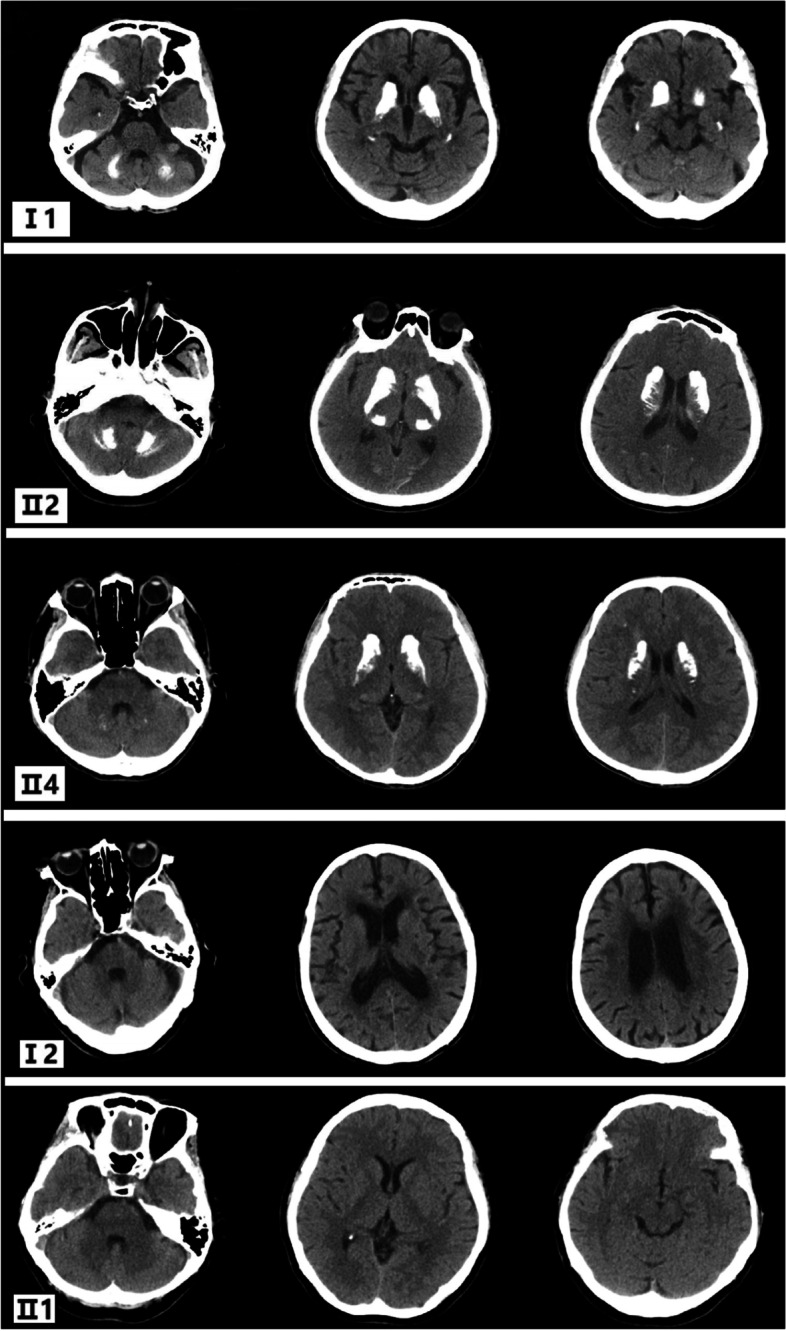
CT report of the proband’s family. The CT scans of his father (I: 1), second sister (II: 2) and younger brother (II:4) showed multiple intracranial calcifications. The CT scan of her mother (I: 2) and elder sister (II: 1) showed no obvious abnormality
